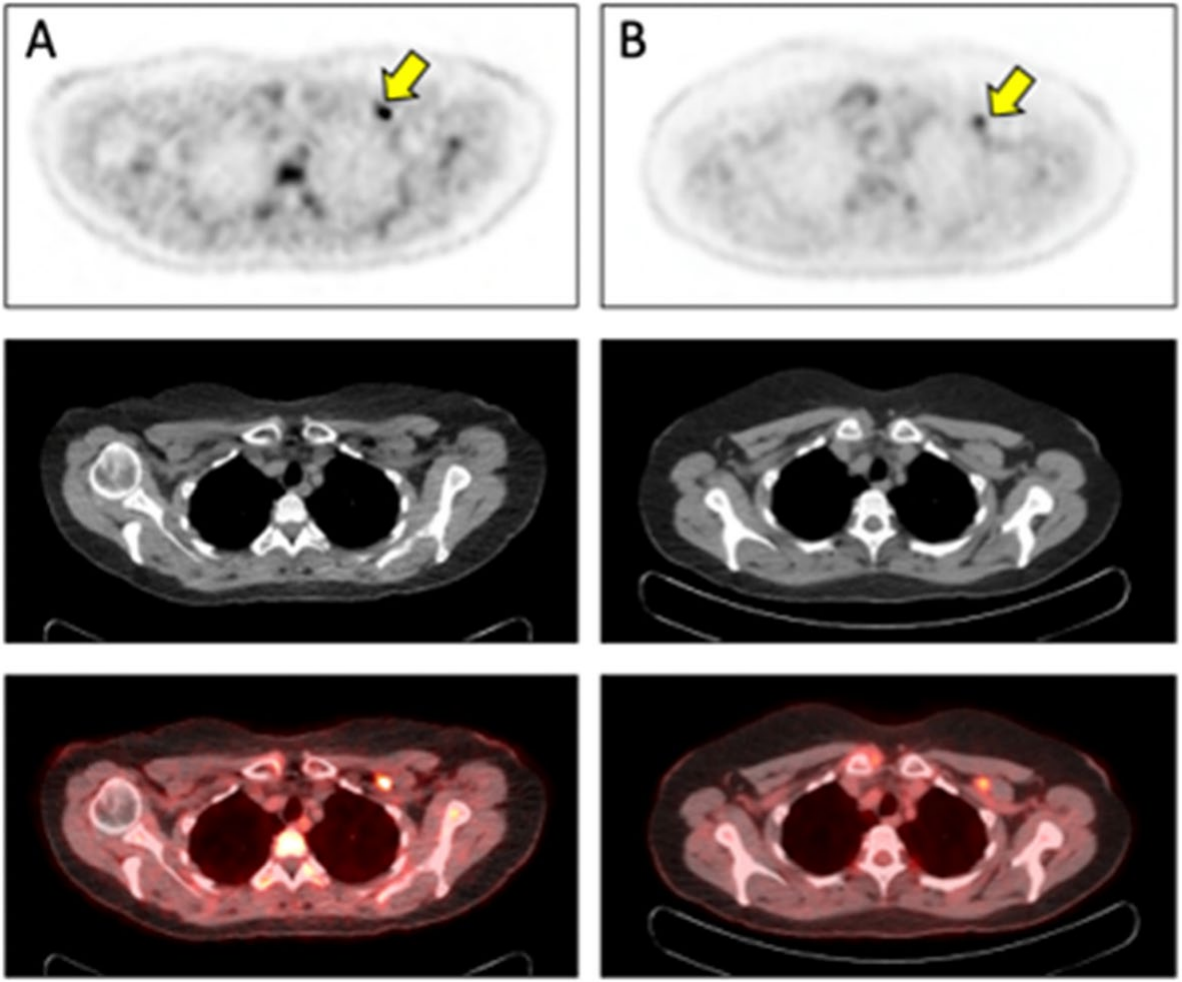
^18^F-Gln-PET/CT (A imaged 67 min post injection) and FDG-PET/CT (B obtained 58 min post injection) images of a patient with ER-expressing breast cancer reveals radiotracer uptake in a left level III lymph node which at pathology demonstrated metastatic ER-positive invasive lobular carcinoma. ^18^F-Gln SUV_MAX_ = 6.4 and FDG SUV_MAX_ = 3.9. High uptake of ^18^F-Gln is reflective of large cellular glutamine pool size and low glutamine catabolism, expected for ER-positive tumors [151]. Modest FDG uptake is also consistent with the modest level of glucose metabolism that is typical for lower grader ER-positive tumors
